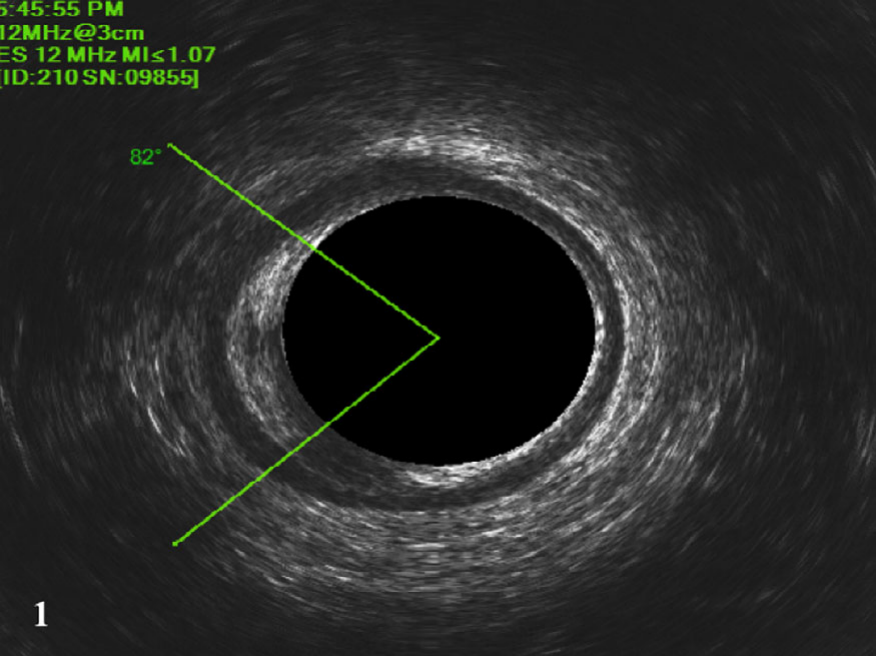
Two‐dimensional endoanal ultrasound showing 82° EAS lesion.
